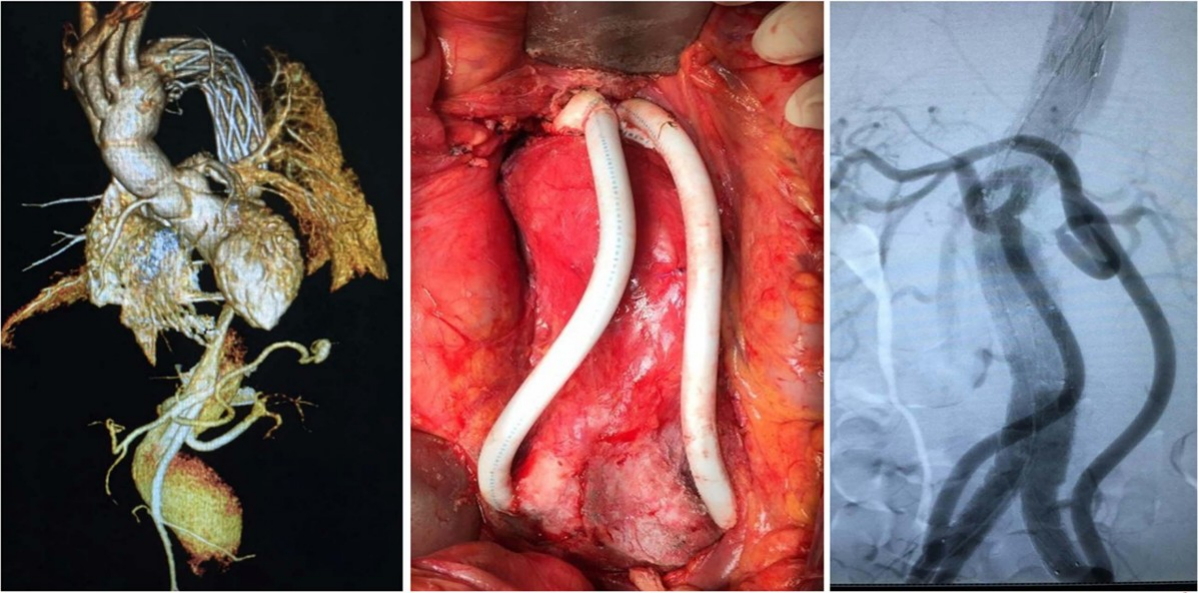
Hybrid treatment for Crawford type III TAAA with Marfan syndrome. Left: the preoperative CTA image of TAAA; Middle: the retrograde revascularization from bilateral CIA; Right: The angiography of DSA for the RVR with bilateral CIA following hybrid treatment.
